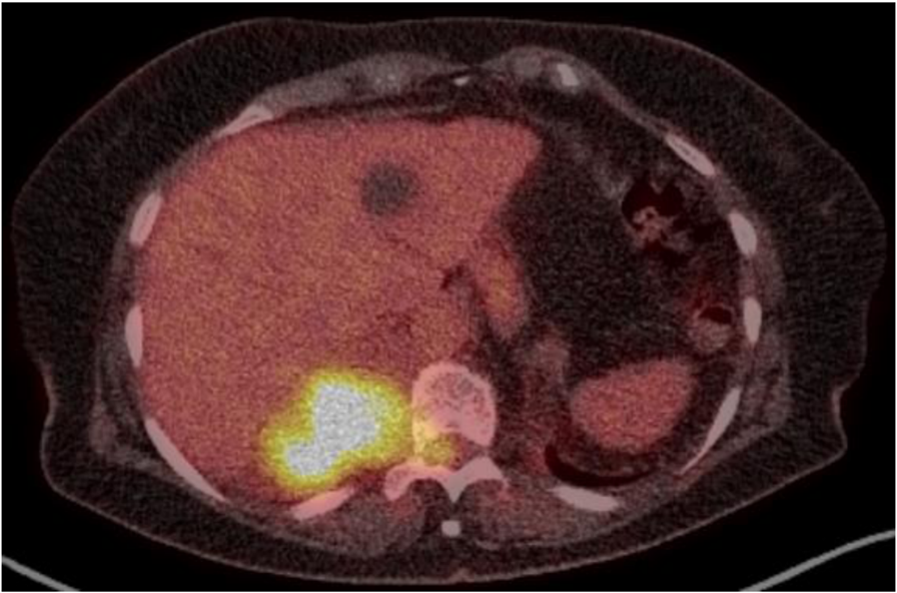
#### O7 Salivary gland tumours: can adding diffusion-weighted imaging to conventional MRI improve accuracy in characterised?
